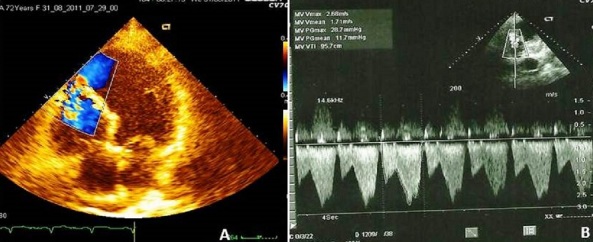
A) échocardiogramme transthoracique, coupe apicale incidence 4 cavités, doppler couleur montrant le flux de la CIV; B) échocardiogramme transthoracique, coupe apicale tronquée, doppler continu montrant un gradient interventriculaire moyen (à travers la CIV) de 11,7 mmHg et maximal de 28,7 mmHg
